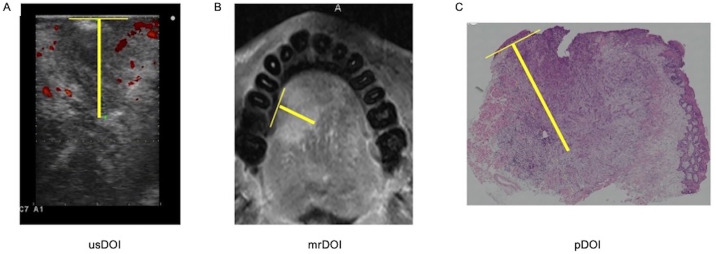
How DOI is measured. Distance from the virtual normal basal mucosa distance to the deepest part of the tumor by ultrasonography (A) and magnetic resonance imaging (B). Distance from the basement of the virtual normal mucosal epithelium to the deepest part of the tumor by pathology (C). usDOI: depth of invasion by ultrasound, mrDOI: depth of invasion by magnetic resonance imaging, pDOI: pathological depth of invasion.
